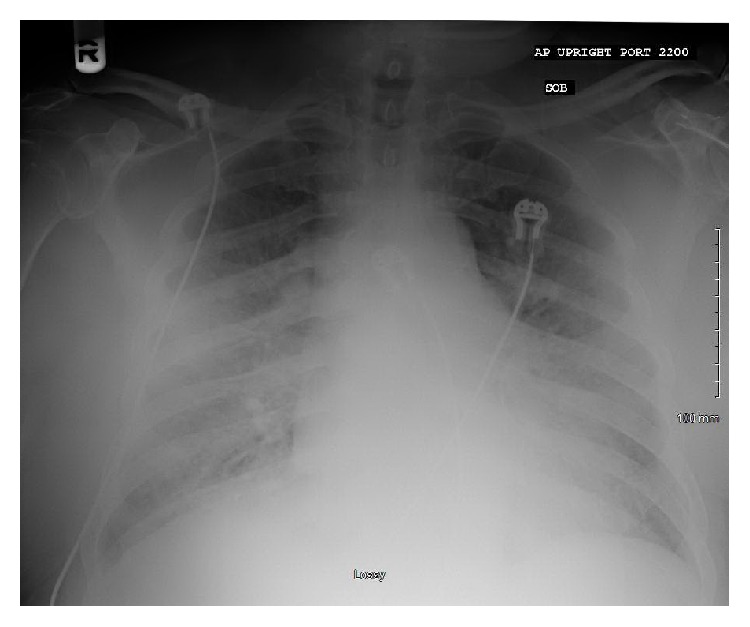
Portable chest X-ray. Minimal cardiomegaly and minimal interstitial pulmonary vascular congestion. Possible small right pleural effusion.
